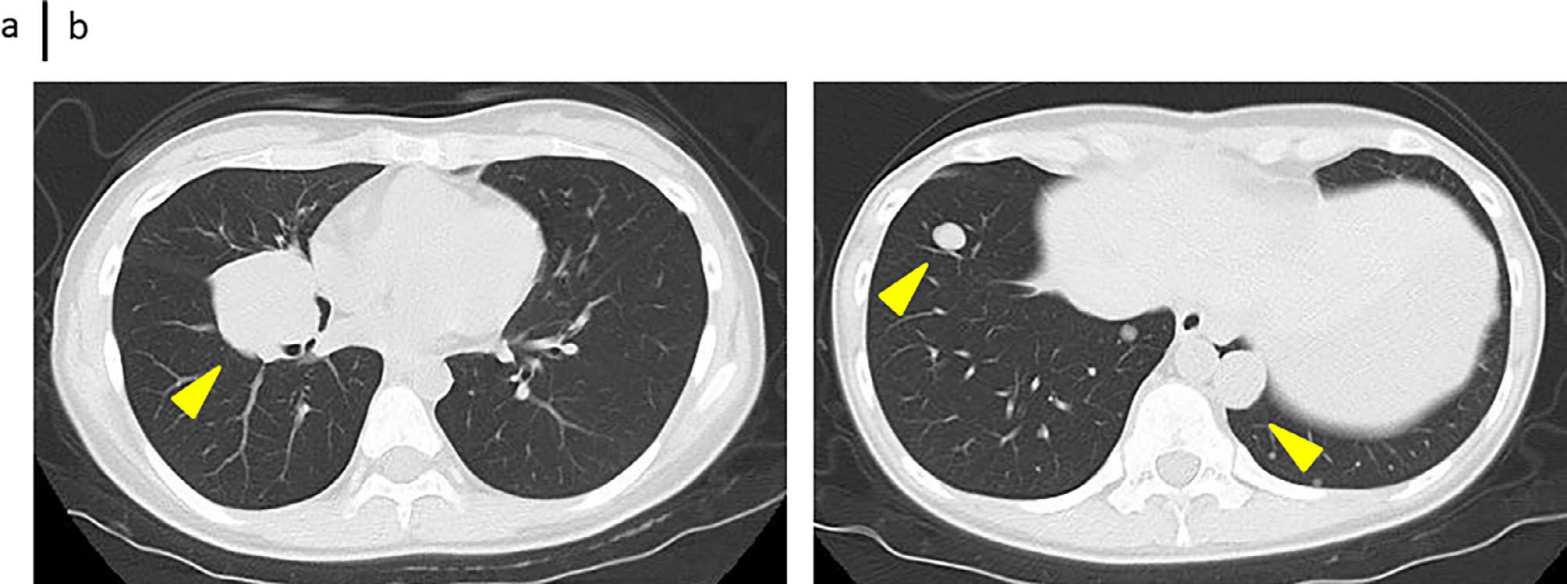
Simple chest CT examination after radiation therapy. (a) The tumor near the right lung hilum did not increase after treatment. (b) The mass in the left lung did not increase after treatment.
